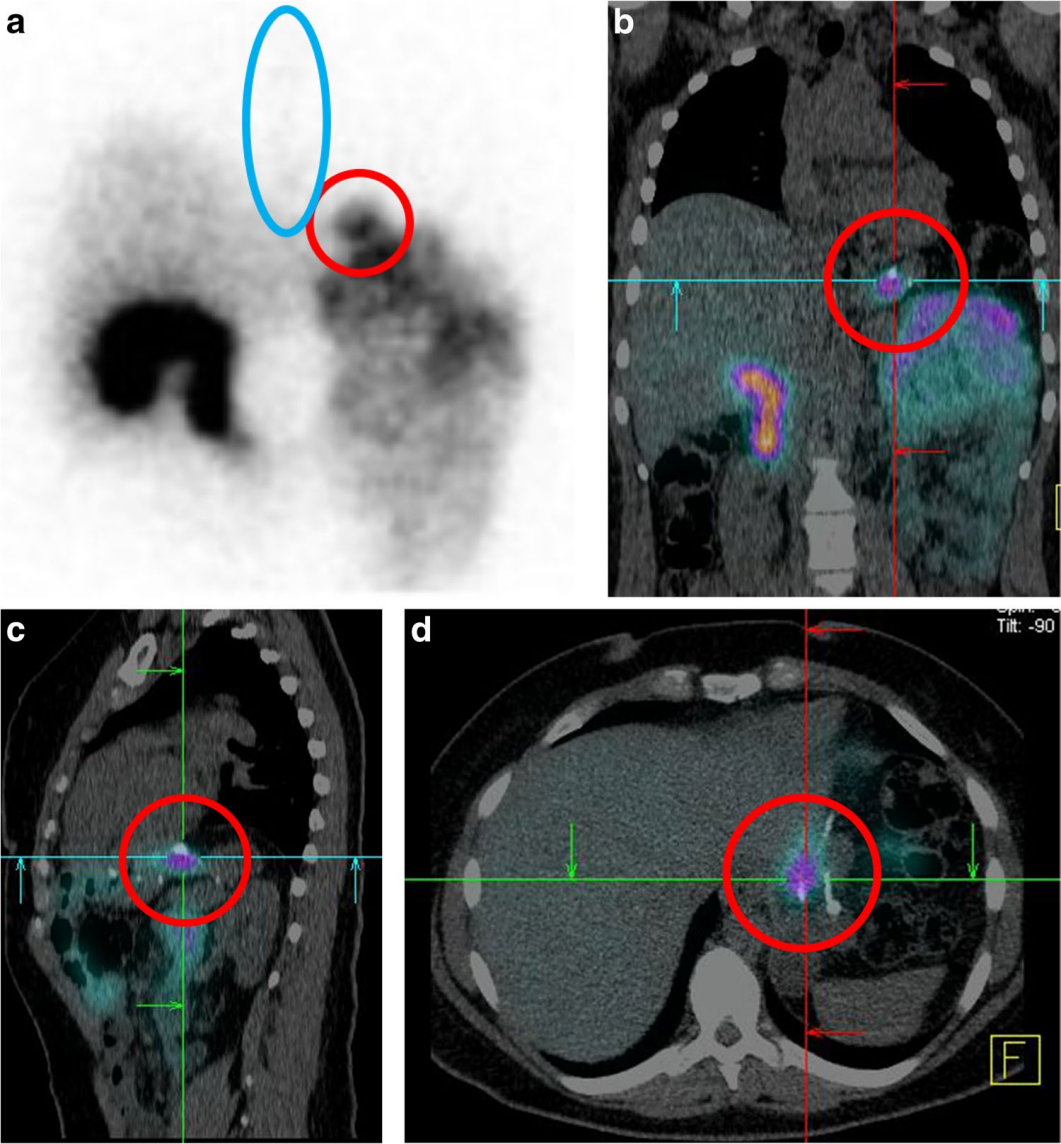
a Scintigraphic image showing reflux into gastric pouch (red circle) and oesophagus (blue circle). b and d: coronal (b), sagittal (c) and axial (d)-fused CT images, confirming localisation of reflux in the gastric pouch (red circle)
